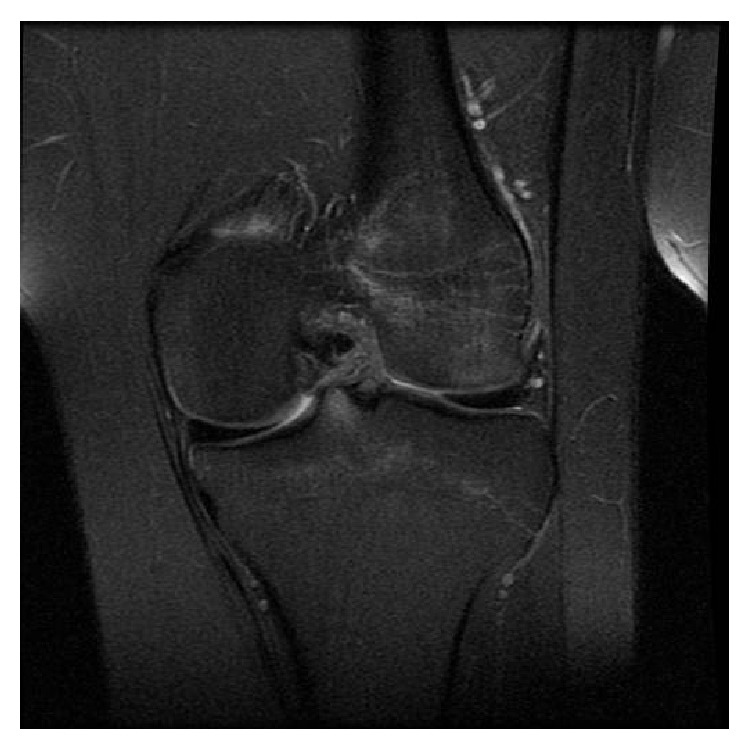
Preoperative coronal MRI scan of the left knee demonstrating a full thickness tear of the anterior cruciate ligament with a typical pattern of bone bruising of the lateral femoral condyle and posterior aspect of the lateral tibial condyle.
